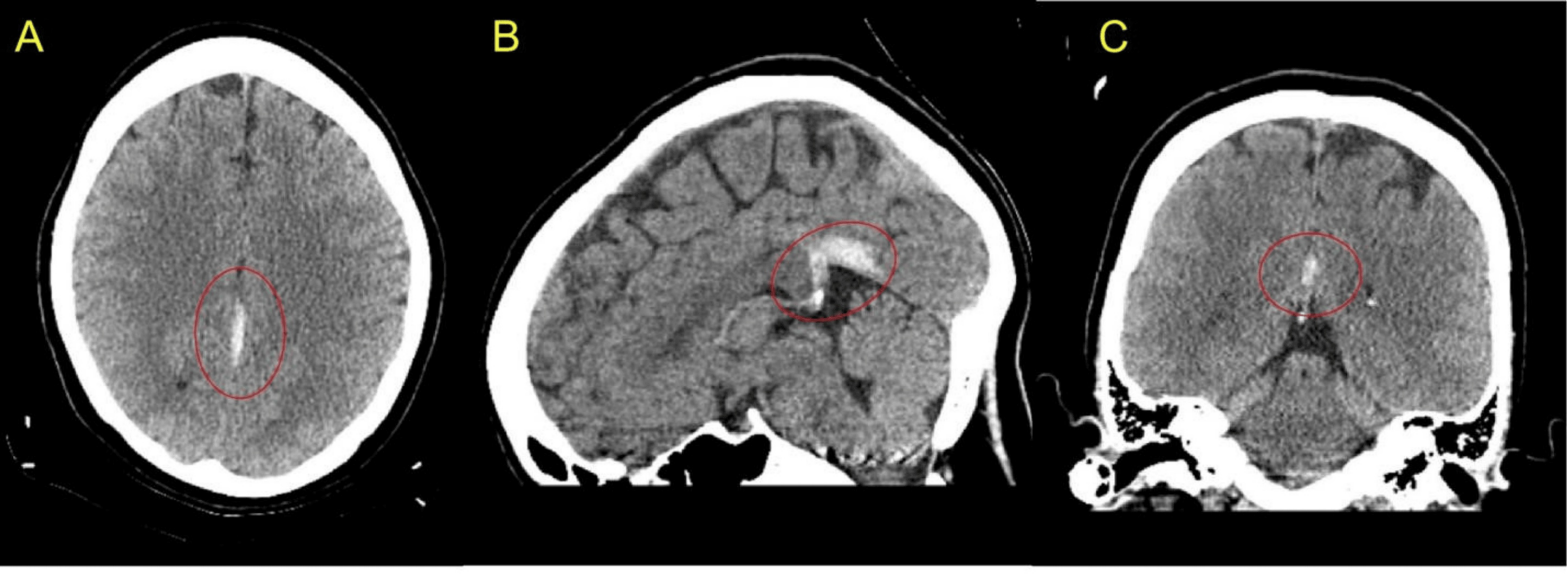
CT images Axial (A), sagittal (B), and coronal (C) images revealed a small acute subdural hematoma measuring approximately 3 mm along the left side of the falx cerebri, extending posteriorly along the left tentorium (encircled in red) CT: computed tomography
